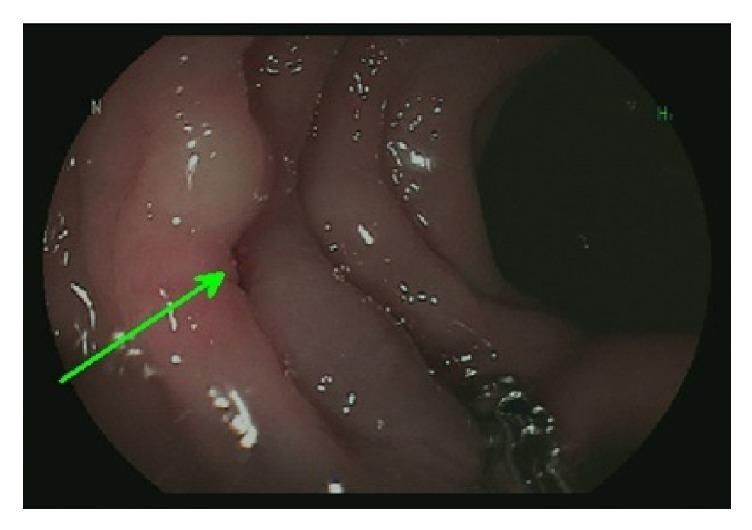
Colonoscopy showing the localized edematous area with bluish discoloration 45 cm from the anus that correlated with the area revealed in the CT scan. Green arrow denotes the edematous area on colonoscopy about 45 cm from anus which likely represents fistula's opening.
